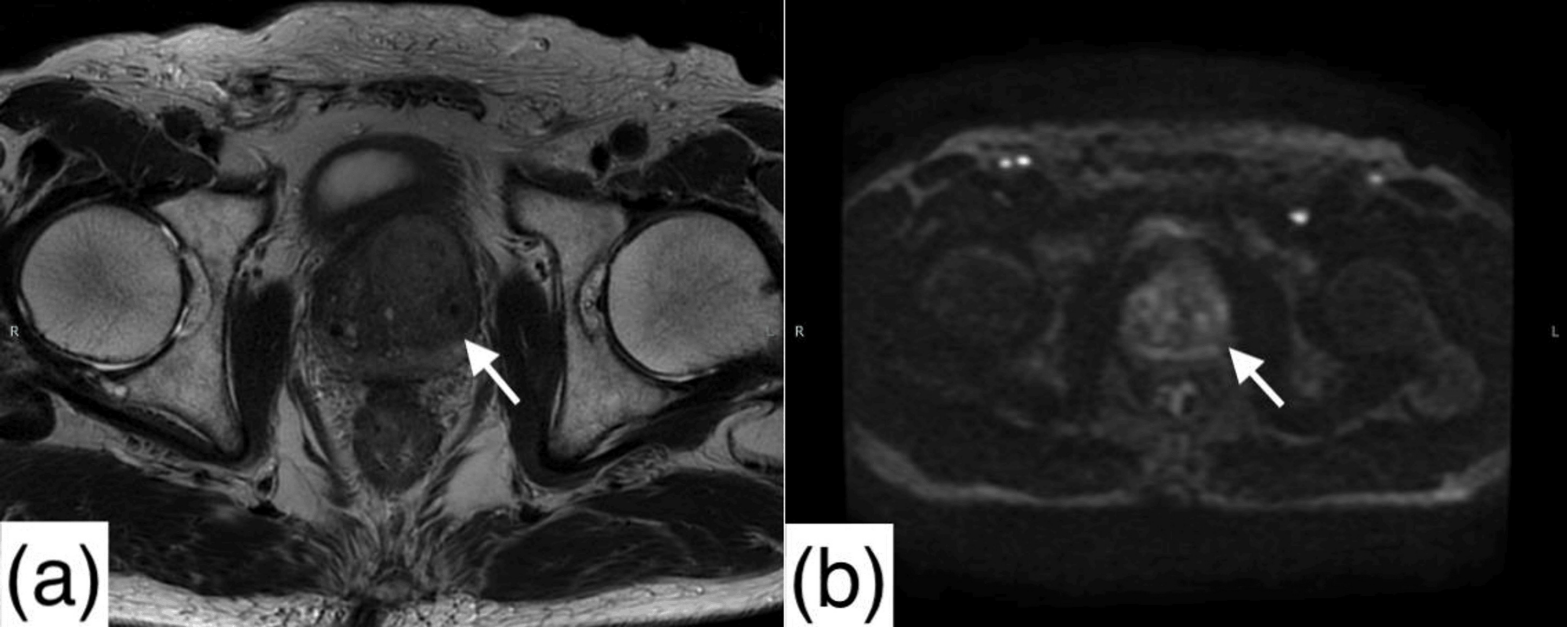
Magnetic resonance imaging of the pelvis (a) T2-weighted sequence reveals no apparent abnormal signal intensity within the prostate (white arrow). (b) Diffusion-weighted sequence reveals no apparent abnormal signal intensity within the prostate (white arrow).
